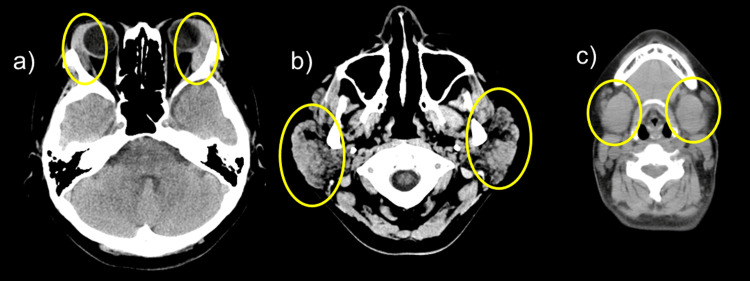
Head and neck CT scan without contrast a) Swelling of the bilateral lacrimal, b) parotid, and c) submandibular glands (yellow circles) CT, computed tomography
